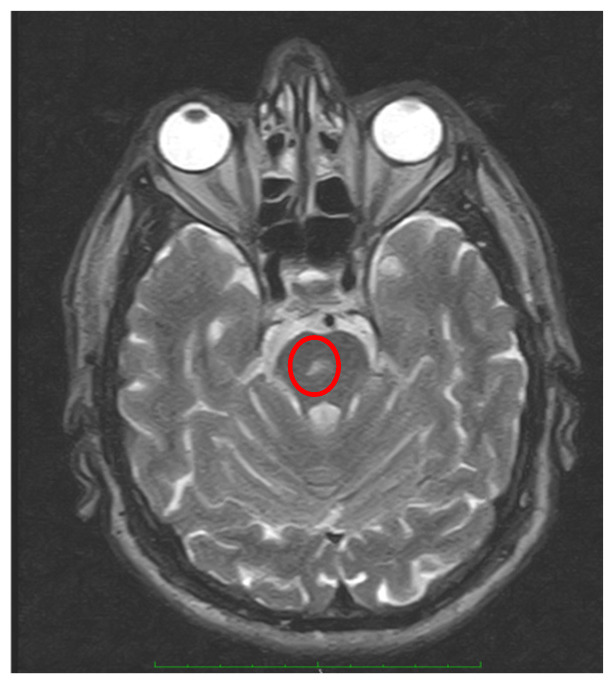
Male, 72, showing at bedside examination a positional bidirectional apogeotropic nystagmus (see Supplementary Material) not responding to repeated repositioning maneuvers. Axial MRI images (T2-weighted with contrast) show a small vascular lesion located at the medial/paramedial right center pontine level (red circle).
